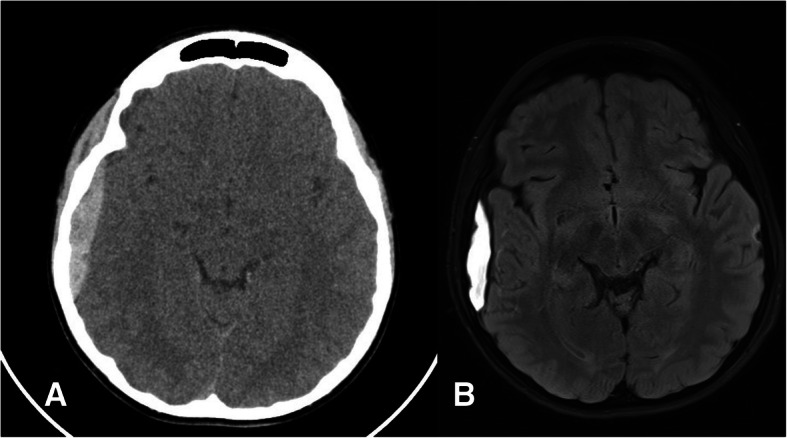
CT scan of an EDH treated conservatively (a) and the MRI performed 2 weeks after trauma (b) showing a slight reduction of the EDH size
